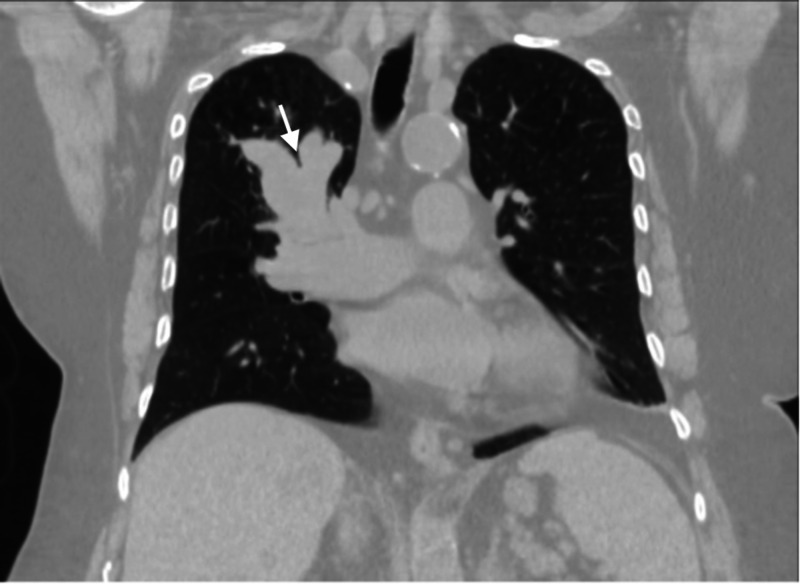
Computed tomography of the chest CT chest shows mass like enlargement of the right upper airway in a finger in glove like pattern (white arrow) suggestive of ABPA. Underlying mass cannot be excluded.
